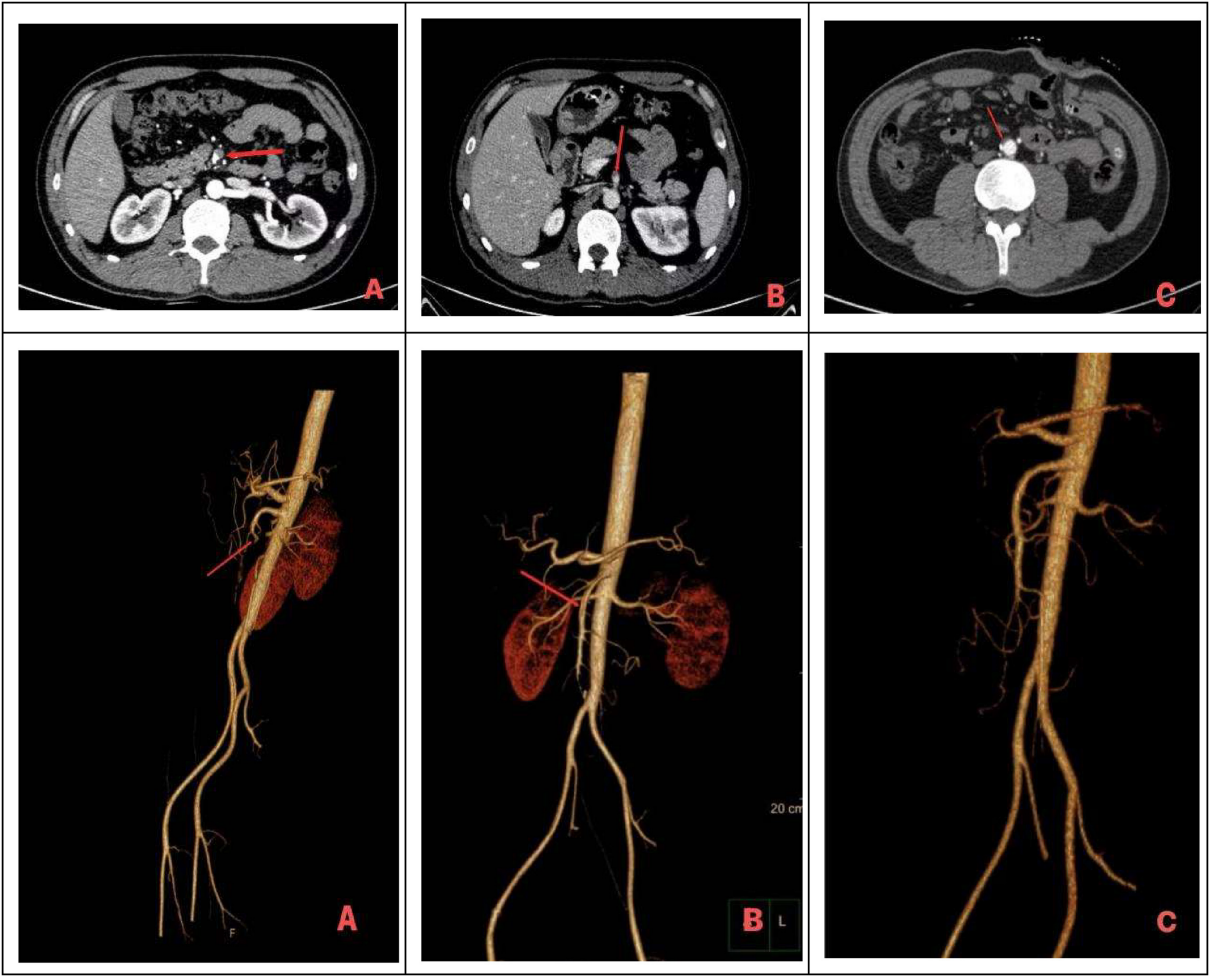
Changes in computed tomography angiography (CTA) and volume rendering (VR) images before and after endovascular and surgical interventions. (A) CTA and VR images obtained on 10 December 2024, before endovascular intervention, demonstrating superior mesenteric artery embolism. (B) Follow-up CTA and VR images obtained on 19 December 2025, after surgical intervention, showing a small amount of mural thrombus in the mid-segment of the superior mesenteric artery, with a marked reduction in thrombus burden compared with panel (A). (C) Follow-up CTA and VR images obtained three months postoperatively (17 March 2025), demonstrating a smooth superior mesenteric artery wall without significant stenosis or focal dilation.
